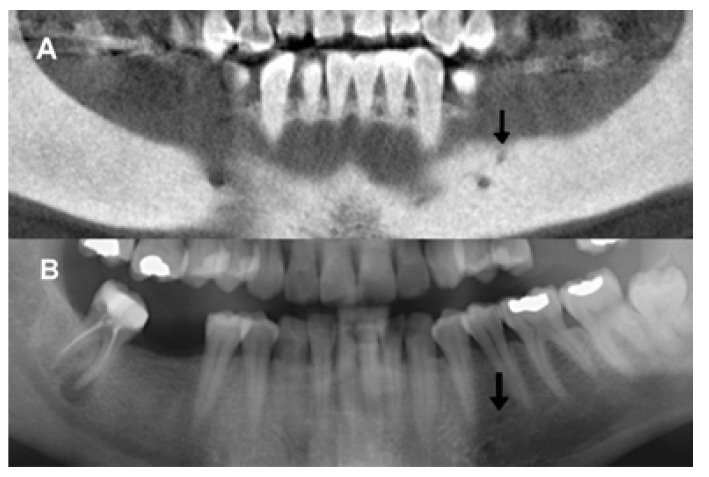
Accessory mental foramen using para-panoramic reconstruction from cone beam CT (A). Accessory mental foramen visualized on corresponding panoramic radiography (B).
